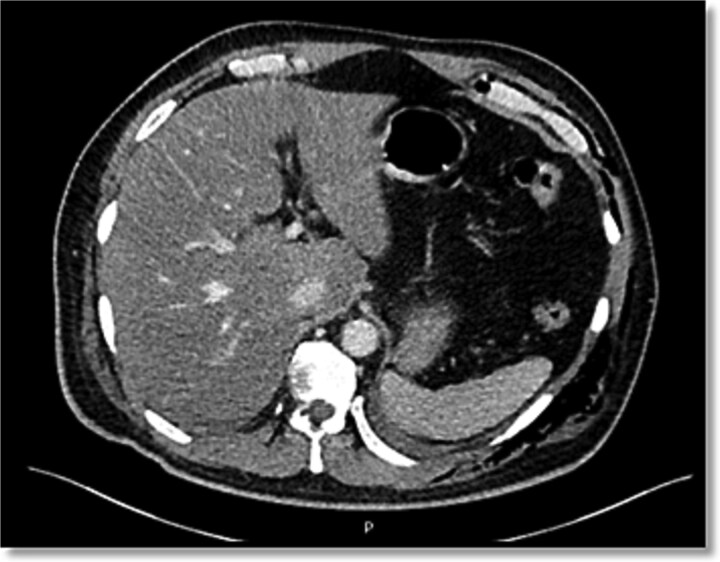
Triphasic abdominal CT, axial view, showing no evidence of hepatic parenchymal injury, preserved vascular integrity, and absence of free fluid or intraperitoneal air.
